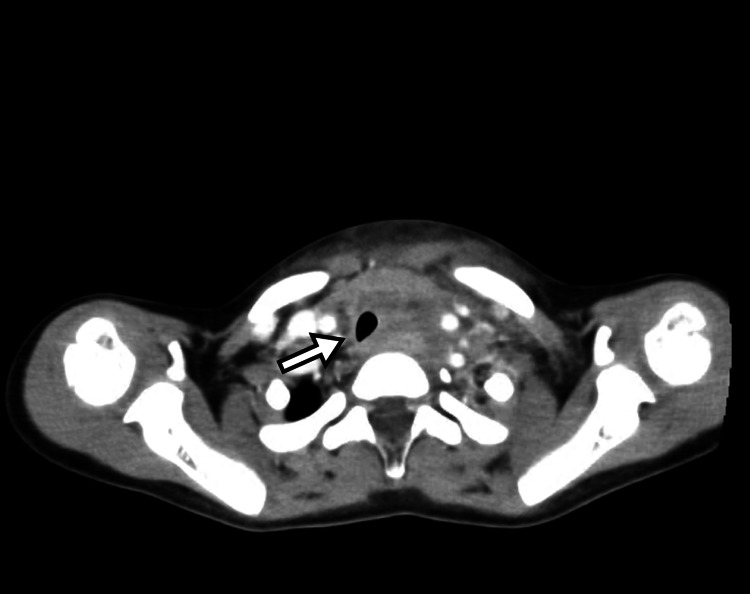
Contrast-enhanced CT on the day of presentation. Contrast-enhanced CT demonstrates displacement of the thyroid gland and trachea to the right, with diffuse soft-tissue swelling extending from the cervical region to the mediastinum. The arrow indicates the rightward-deviated trachea.
